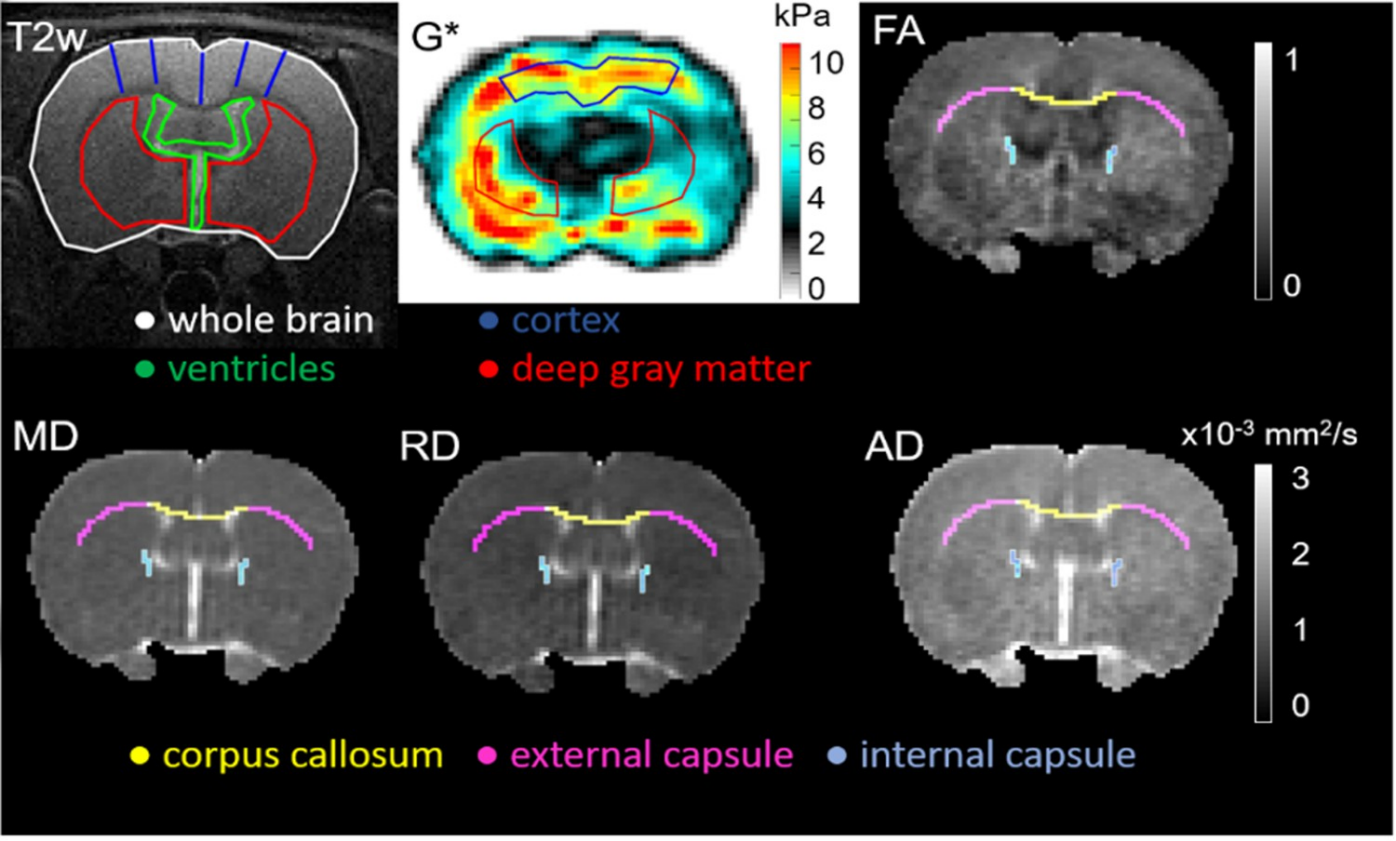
Typical images/maps obtained with this MRI protocol. Anatomical T2 weighted (T2W) image; shear modulus (G*) map, as measured by MRE at 800 Hz; fractional anisotropy (FA), mean diffusivity (MD), axial and radial diffusivity (AD and RD) maps, as measured with DTI (Bregma -0.72 mm (29)). Anatomical images were used to measure the cross-sectional areas of the ventricular system (green), whole-brain (white), and deep gray matter (red), along with the cortical thickness in the middle slice acquired (navy blue). The cortical thickness was averaged over 5 locations on the “roof” of the lateral ventricles. The magnitude of the complex shear modulus (G*) was calculated for the cortex (navy blue) and deep gray matter (red). FA, MD, RD and AD were obtained for the corpus callosum (yellow), external (pink) and internal (purple) capsules.
